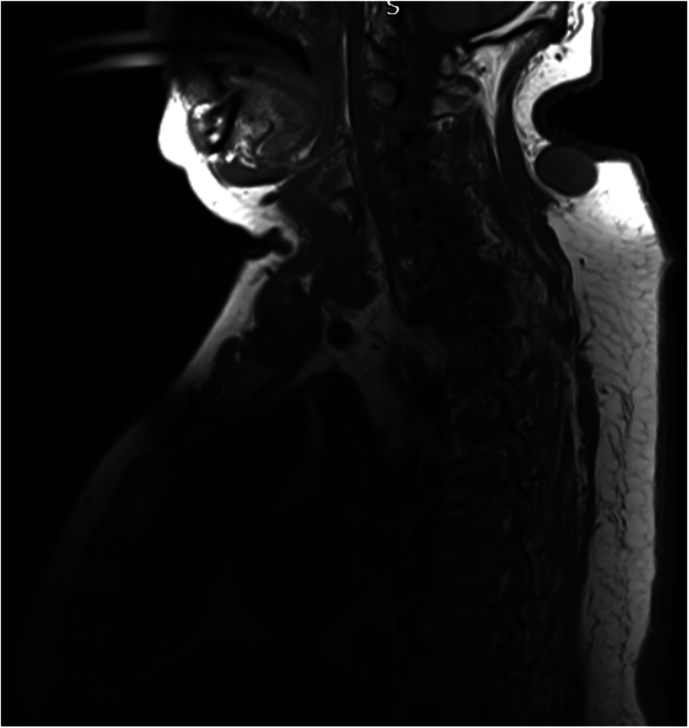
Sagittal view of cervical MRI. Charcot spinal arthropathy noted at the levels of C7-T1 with disc narrowing and edema.
